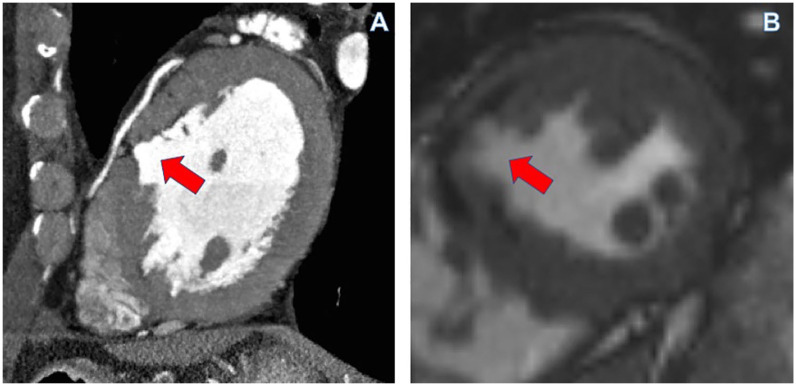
(A) Enhanced cardiac CT sagittal view shows focal aneurysm in the mid septal segment of the left ventricle (arrow) with associated minimal fatty infiltration and (B) cardiac MR short axis view confirms the presence of focal aneurysm as demonstrated on the cardiac CT.
